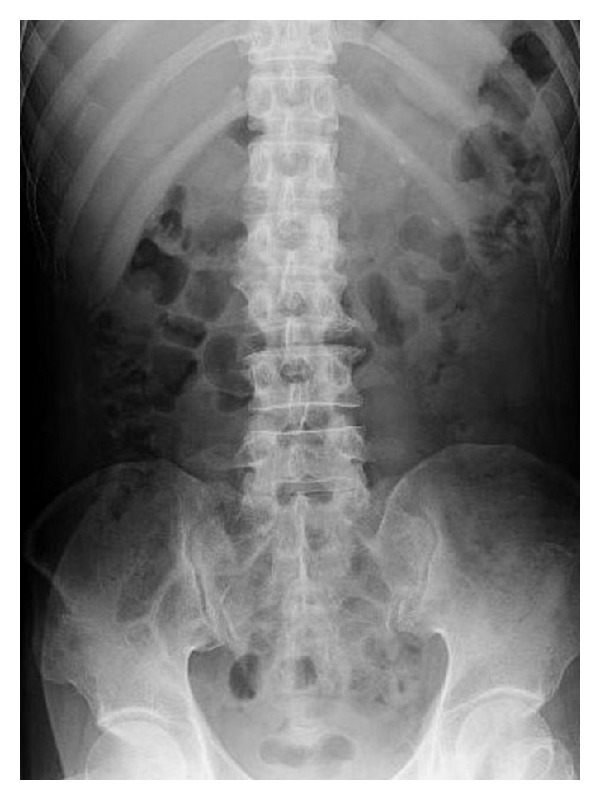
Preoperative abdominal X-ray.
